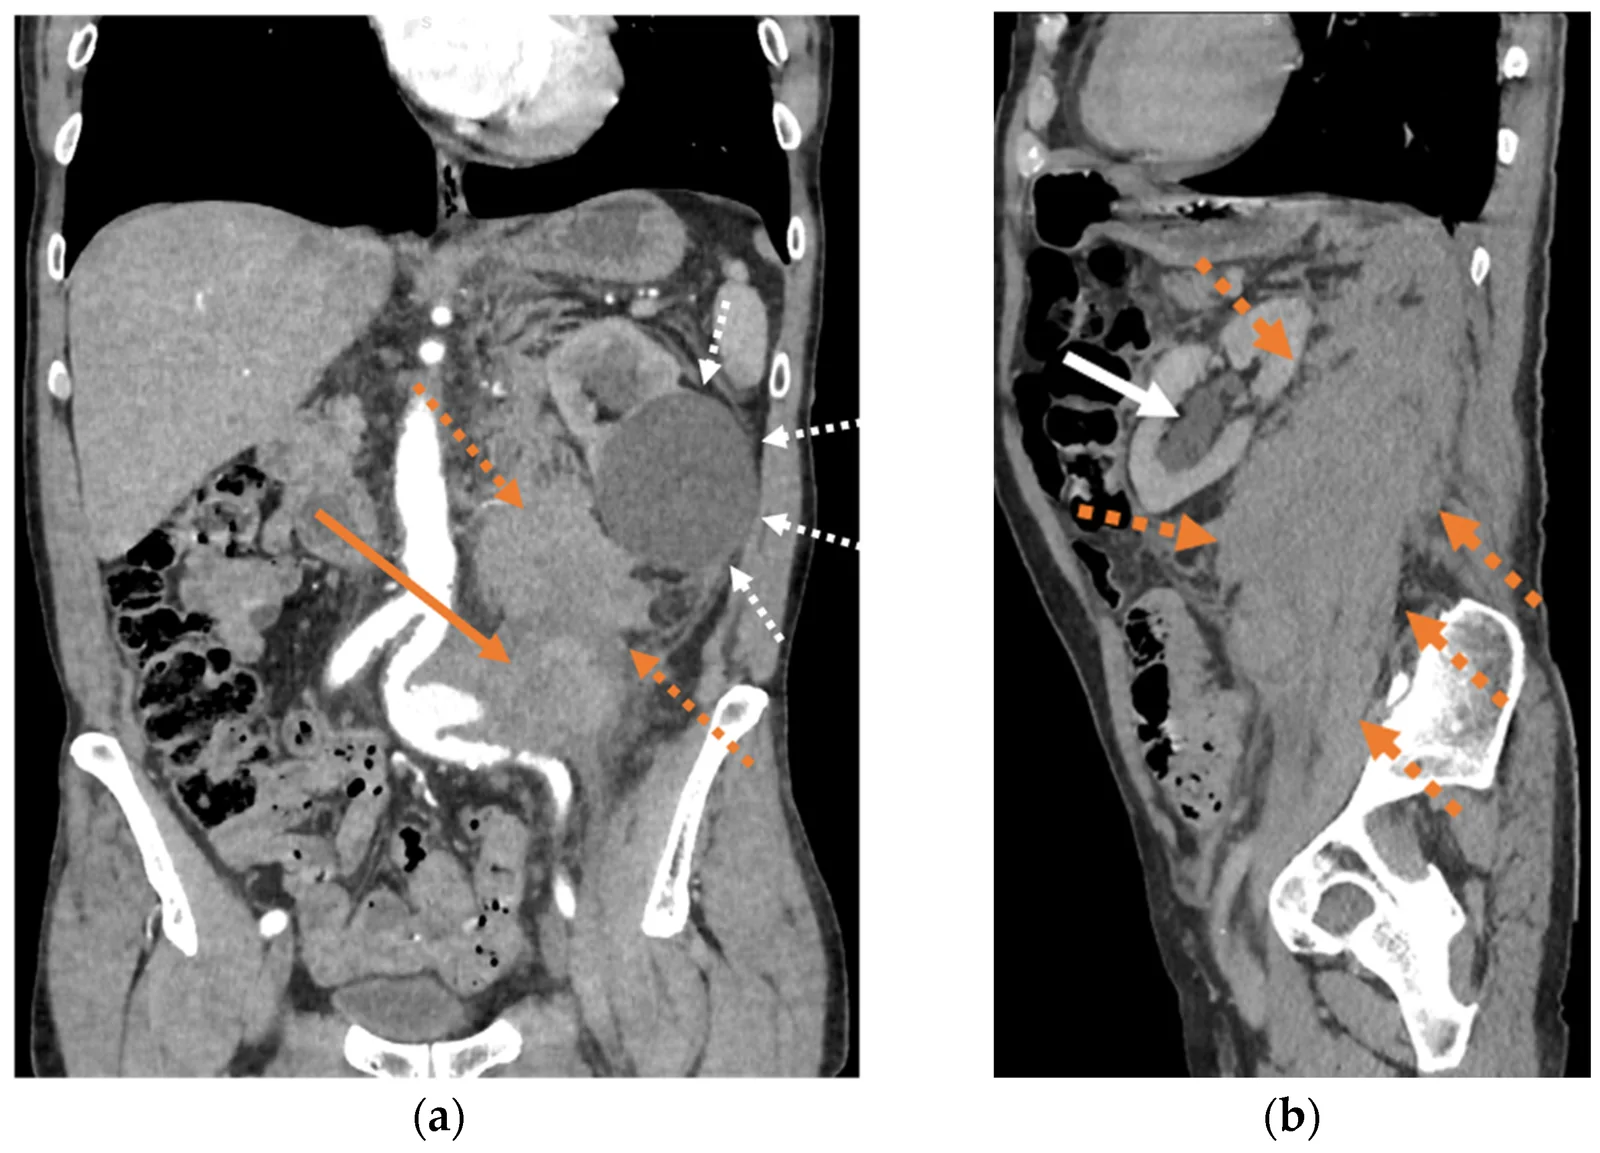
Contrast-enhanced computed tomography of the abdomen and pelvis obtained in the emergency department. (a) Arterial-phase coronal image showing a fissured saccular aneurysm of the left common iliac artery, measuring approximately 4.0 × 2.5 × 3.0 cm (orange arrow), with active contrast extravasation and an associated retroperitoneal hematoma measuring approximately 20 × 9 × 6.5 cm (orange dashed arrows). A large left renal cortical cyst, measuring approximately 7.3 × 6.0 × 7.0 cm, is indicated by dashed white arrows. (b) Delayed-phase sagittal image demonstrating the craniocaudal extent of the retroperitoneal hematoma (orange dashed arrows) and its mass effect on the left kidney and adjacent abdominal structures, resulting in grade II–III left hydronephrosis (white arrow).
